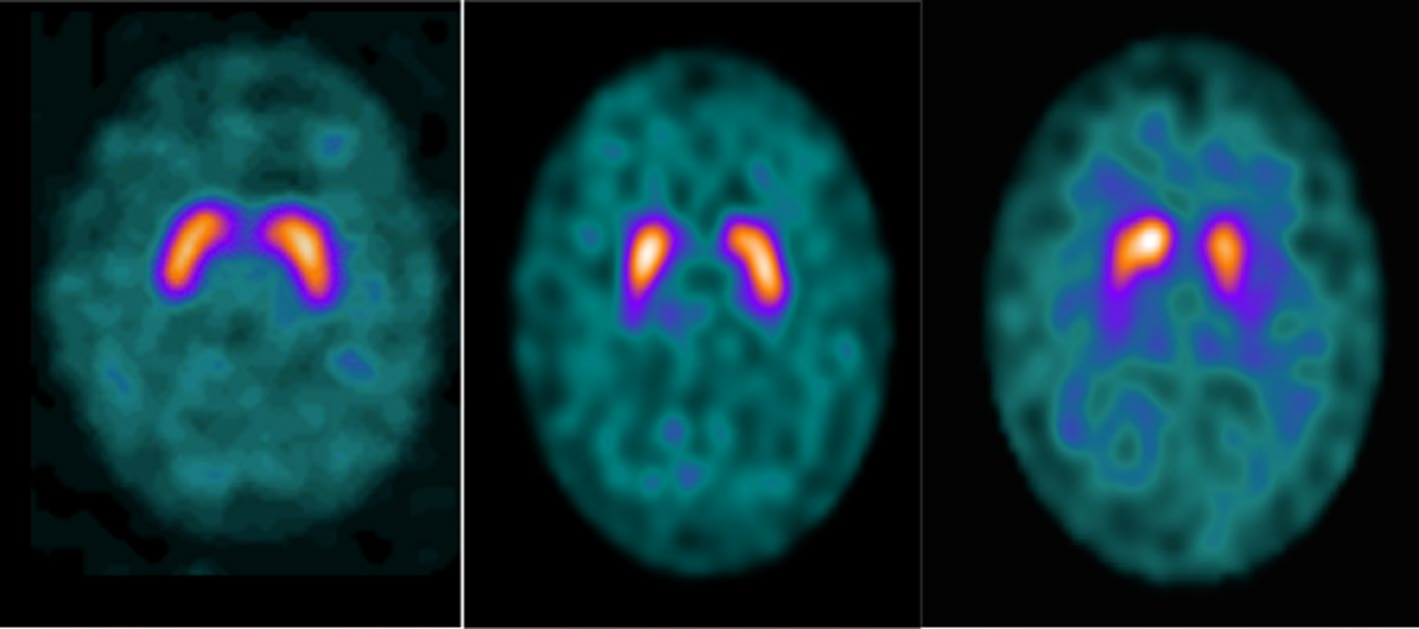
SWEDD (left), borderline (middle) and abnormal (right) [^123^I]Ioflupane (DaTSCAN) SPECT Scans. SWEDD demonstrates bilateral inverted comma shape representing the caudate nucleus (at the top) and putamen (at the bottom). Borderline image shows a suspicious “weak comma” on the right side of the brain scan but had normal SBR values. The abnormal scan shows an obvious left-sided “full stop”-shaped binding with diminished SBR in this area
